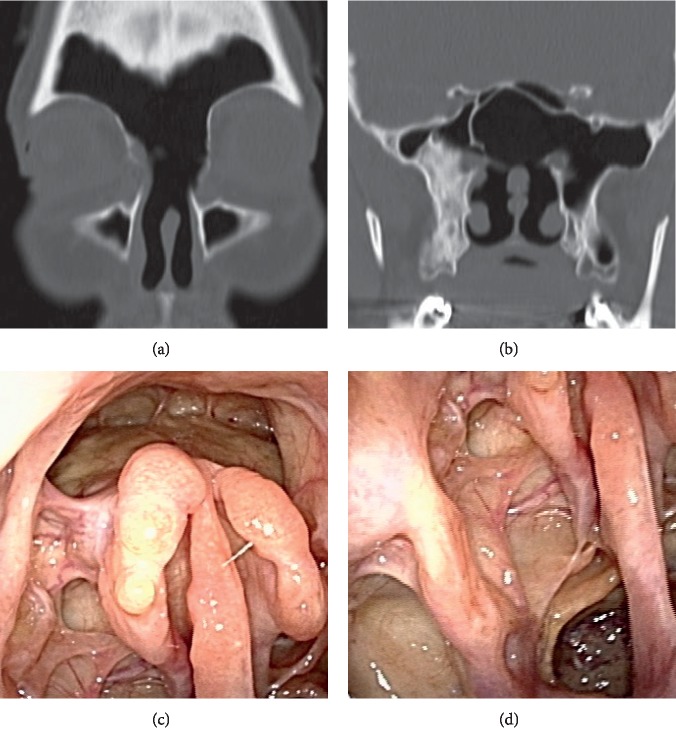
Continued remission in Case 2. (a, b) Computed tomography image findings of Case 2 after the second surgery. (c, d) Pathological findings of the paranasal cavity in Case 2 after the second surgery. There is no apparent evidence indicating disease recurrence.
